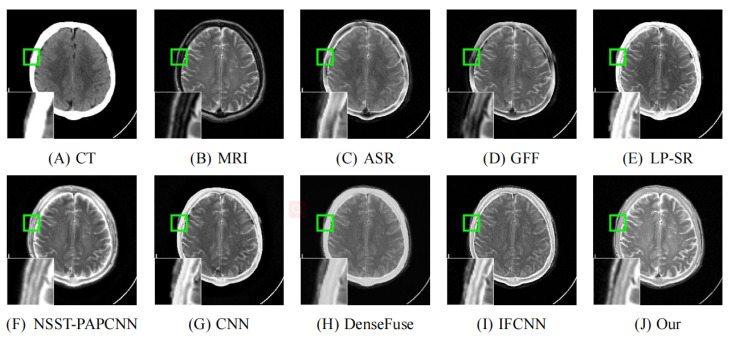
Fusion images of “Acute stroke speech arrest-16” by various methods. From (A) to (J): CT, MRI, ASR, GFF, LP-SR, NSST-PAPCNN, CNN, DenseFuse, IFCNN, Our proposed method.
